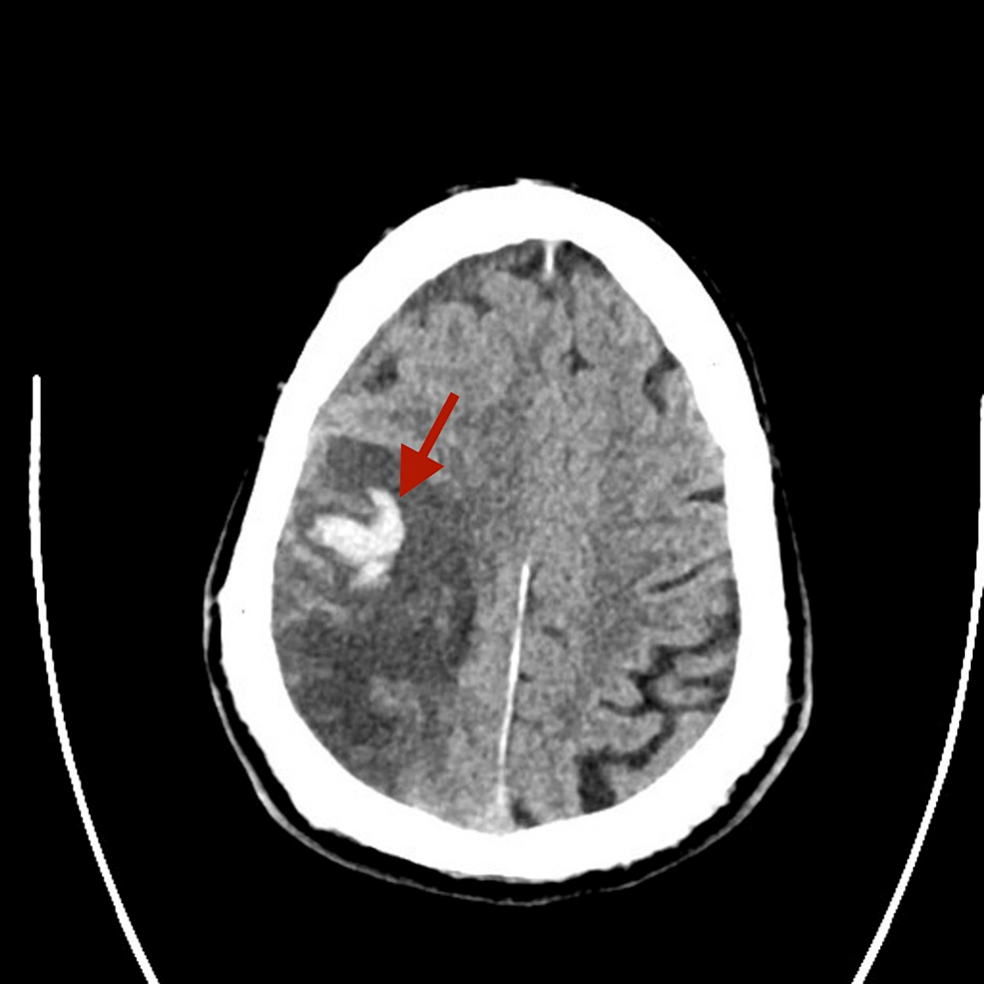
Repeat non-contrast CT brain done on day 13 post clinical deterioration revealing hemorrhagic transformation of the right middle cerebral artery (MCA) stroke.
